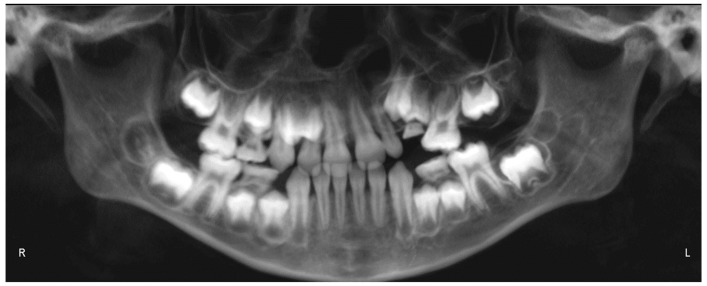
A panoramic view derived from CBCT volume depicting the ankylosed and submerged primary maxillary left second molar.
